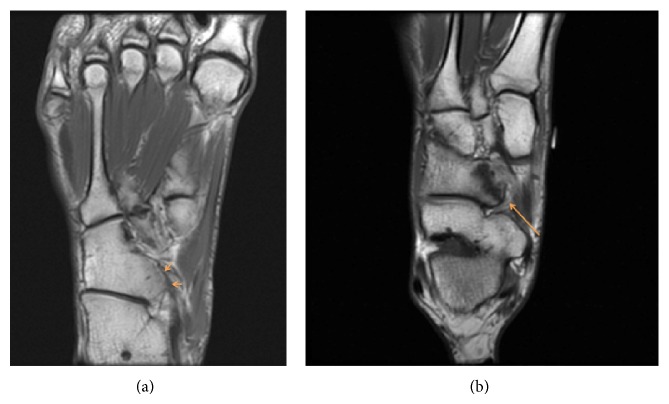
(a)-(b) Coronal T1 weighted unenhanced MR images of the right foot in a 17-year-old male show an elongated posterior medial process of the cuboid (arrows, (a)) that articulates abnormally with the plantar lateral aspect of the navicular (arrow, (b)), consistent with cuboid-navicular coalition.
